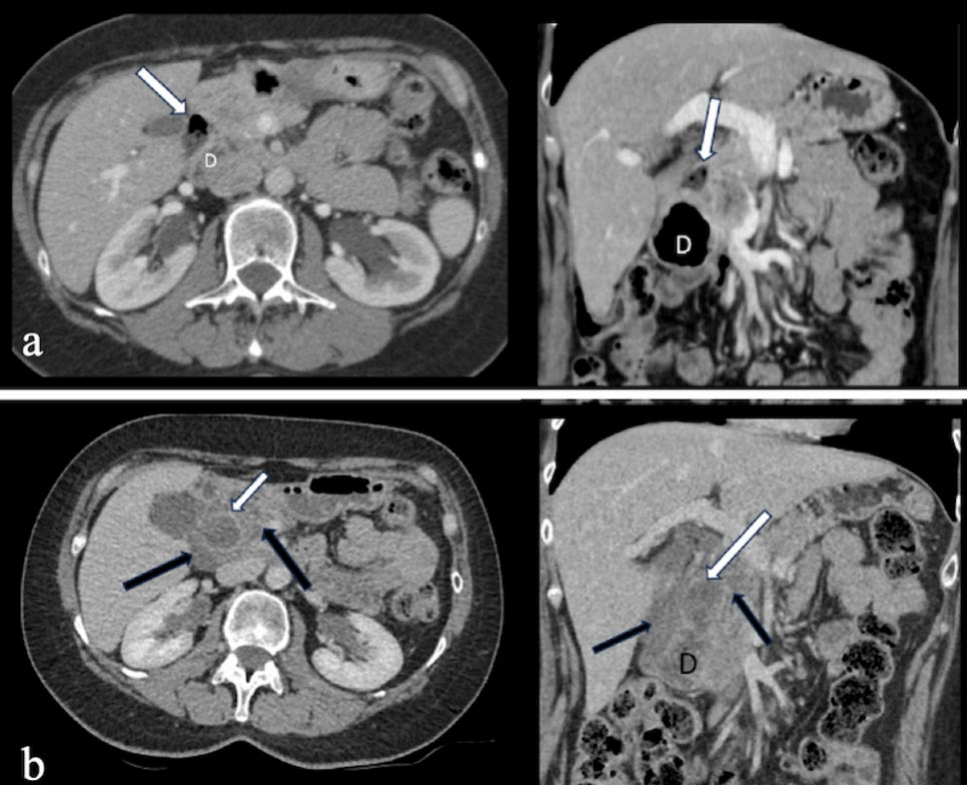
Axial and coronal IV contrast-enhanced CT images of a 61-year-old female patient. a. CT images obtained five years prior show a duodenal diverticulum (white arrows). b. Recent CT images reveal significant duodenal wall thickening, hyperenhancement, periduodenal heterogeneity, and fluid collections (black arrows), findings consistent with duodenal diverticulitis
